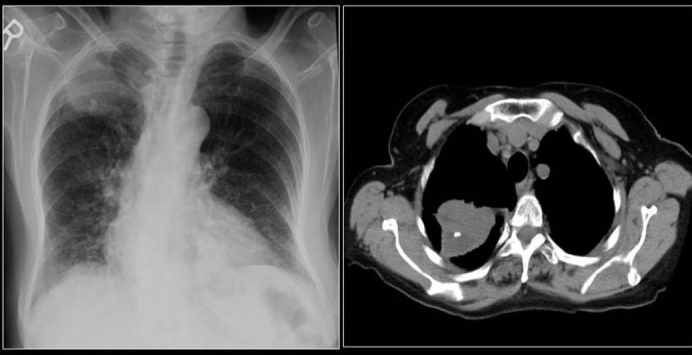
A chest radiograph and axial CT scan shows a dense nidus of central calcification in an adenocarcinoma of the lung
